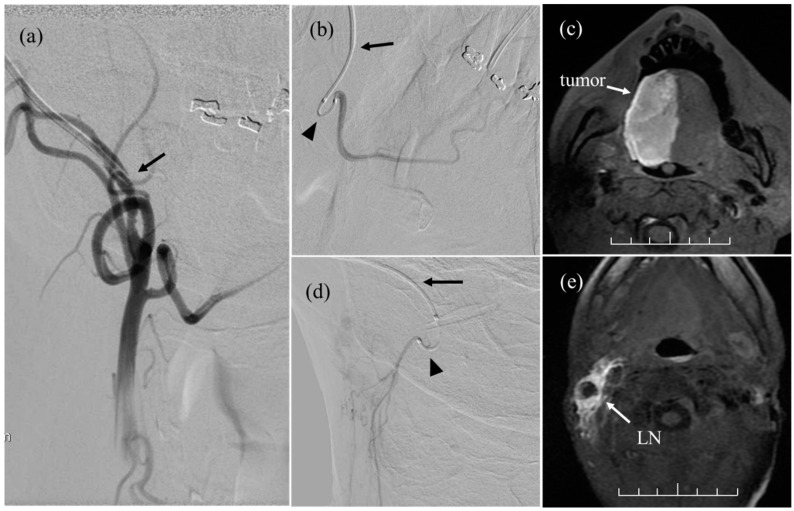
(a) External carotid arteriography obtained by administering contrast media via the external carotid arterial sheath (ECAS) on digital subtraction angiography (DSA). Arrow: tip of ECAS. (b) Superselective lingual arteriography via a steerable microcatheter through the ECAS on the DSA. Arrow: tip of ECAS; arrowhead: a steerable microcatheter. (c) Magnetic resonance image showing the injected contrast agent via the right lingual artery. (d) Arteriography of a branch from the ECA to the metastatic lymph nodes on the DSA. (e) Magnetic resonance image showing the injected contrast agent via the direct branch from the ECA. Scale bar.
